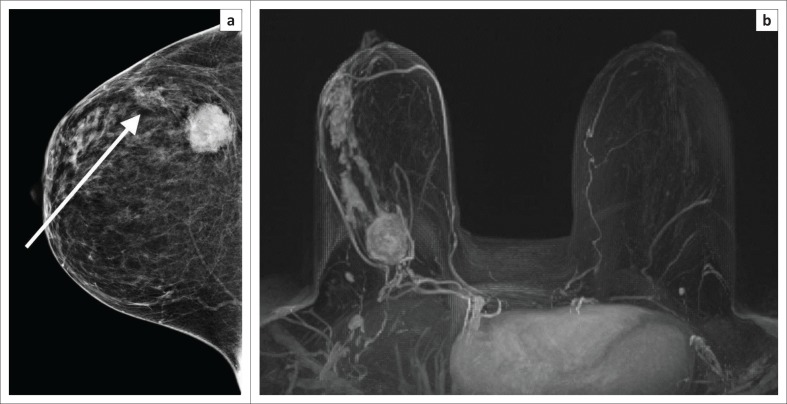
(a) Mammogram of a 56-year-old woman with a large cancer in the posterior outer aspect of the right breast. There is asymmetry and subtle distortion anterior to the mass (white arrow). This is suspicious for intra-ductal extension (associated ductal carcinoma in situ). The extent on mammogram measures 6 cm. (b) Post-contrast magnetic resonance imaging axial maximal intensity projection (MIP) shows extensive segmental non-mass enhancement extending all the way from the posteriorly situated mass to the retroareolar space. Extent is 11 cm.
